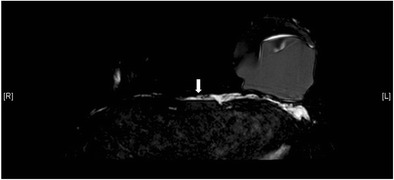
Chest wall extension of PAAG in a patient with history of left breast augmentation by PAAG injection and silicone gel-filled implant. Extension of PAAG to chest wall is clearly shown on this T2-weighted, fat-saturated MR axial image. Silicone is of low signal while PAAG is of high signal on this sequence. Medial extension of PAAG to pre-sternal region is noted (arrow)
